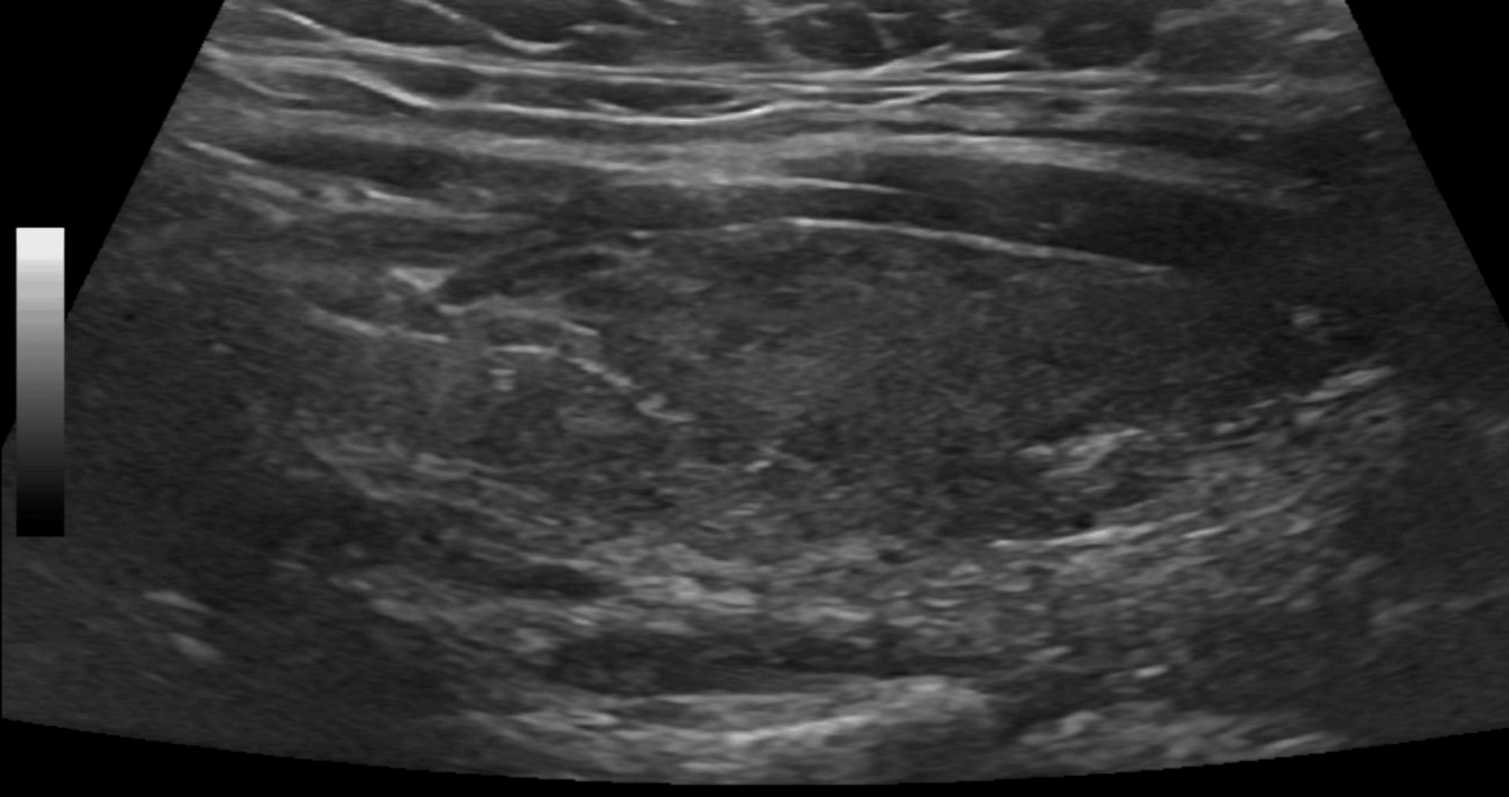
Longitudinal ultrasound image of the right thyroid lobe showing homogeneous echotexture without nodules
